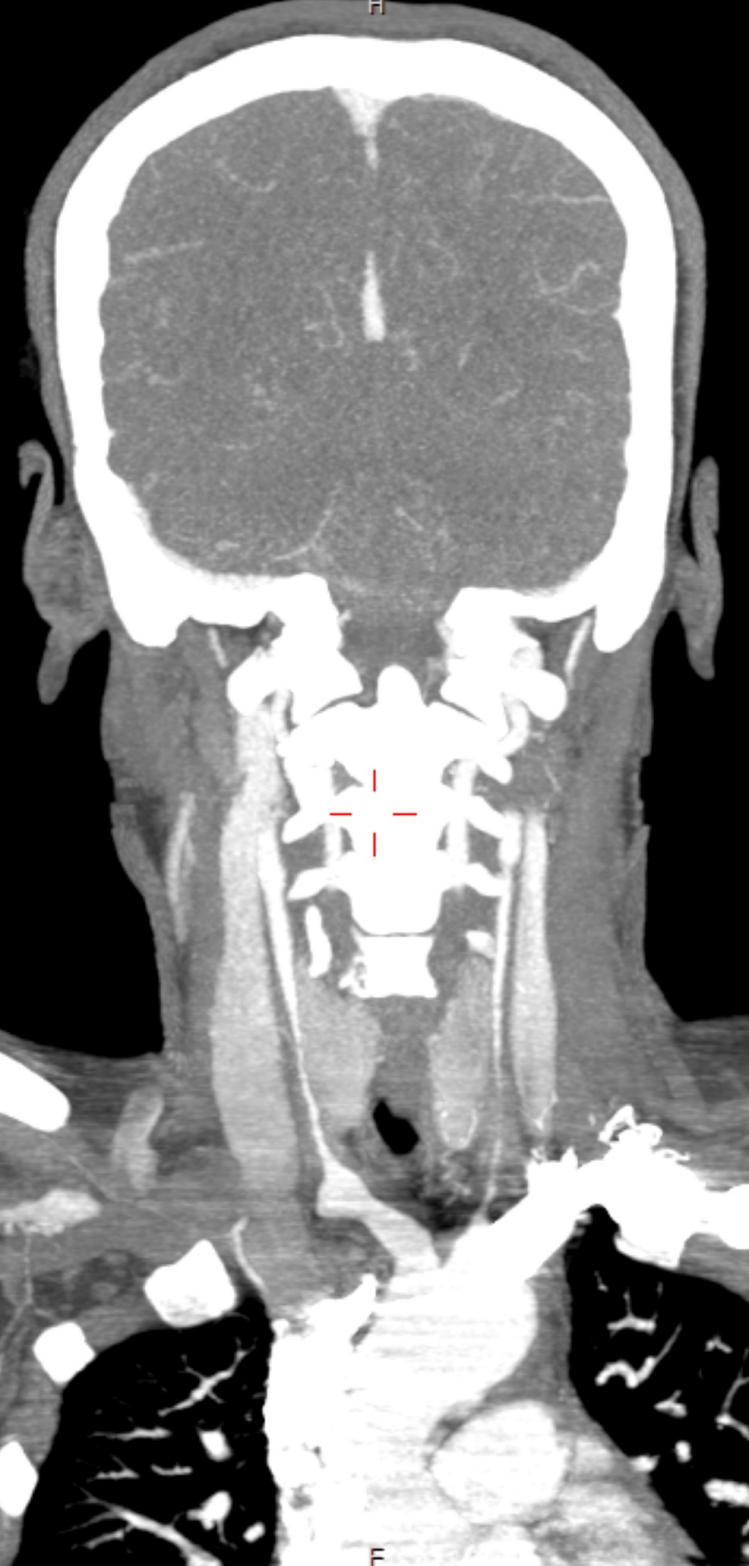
A computed tomography angiography of the head and neck showing severely attenuated bilateral common carotid arteries from their origin, as well as smooth wall thickening of the aortic arch and its branches
